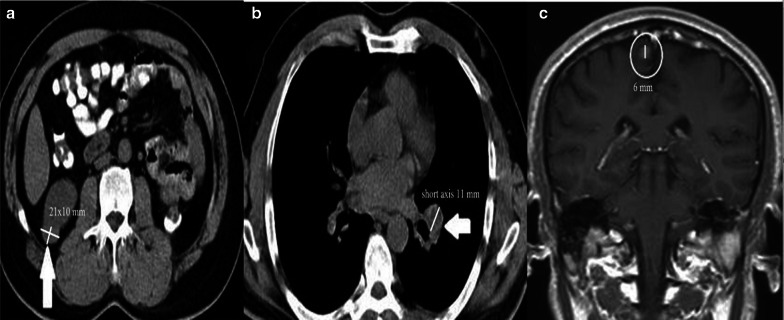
52- year old male patient diagnosed with metastatic renal cell carcinoma. a, b Axial CT images show hypodense malignant lesion (arrow) in the lower pole of the right kidney and pathological lymph node in the left hilar region (arrow). c Coronal post-contrast T1 weighted MR image shows metastasis in the right parietal lobe (circle). Chemotherapy was started
